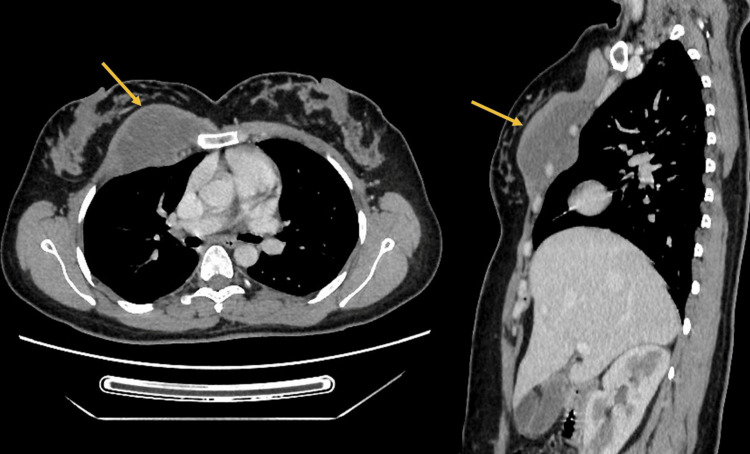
Axial (A) and sagittal (B) sections of the post-contrast chest CT scan Images showing the enhancement of the anterior chest wall mass (yellow arrow)
